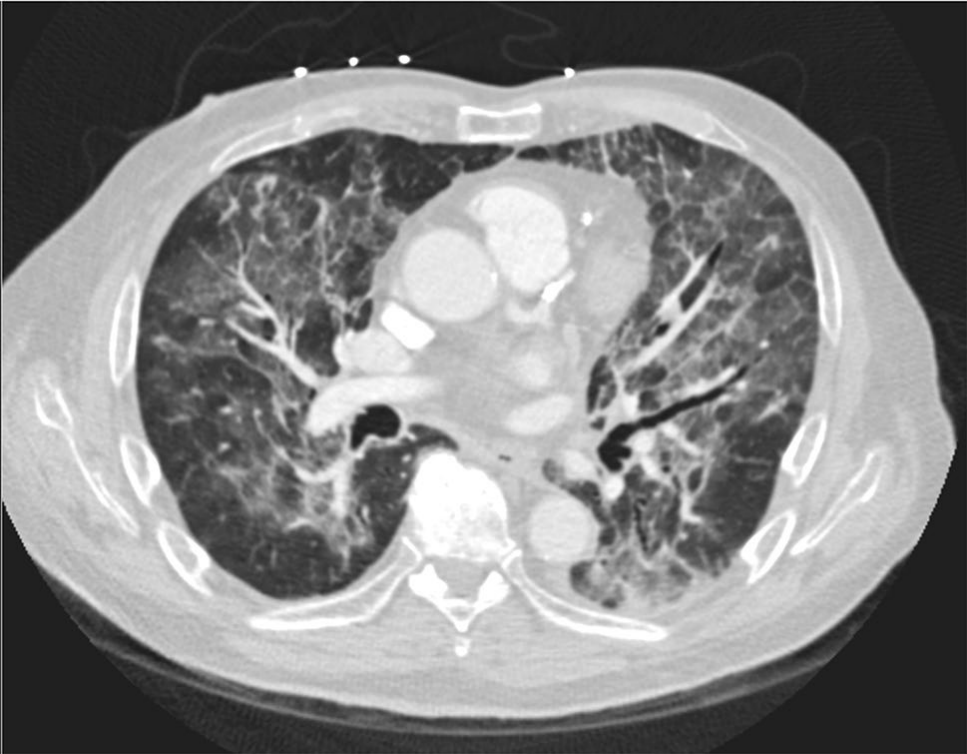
Computerized Tomography (CT) scan demonstrating bilateral ground glass opacities with concurrent diffuse consolidative and nodular opacities
